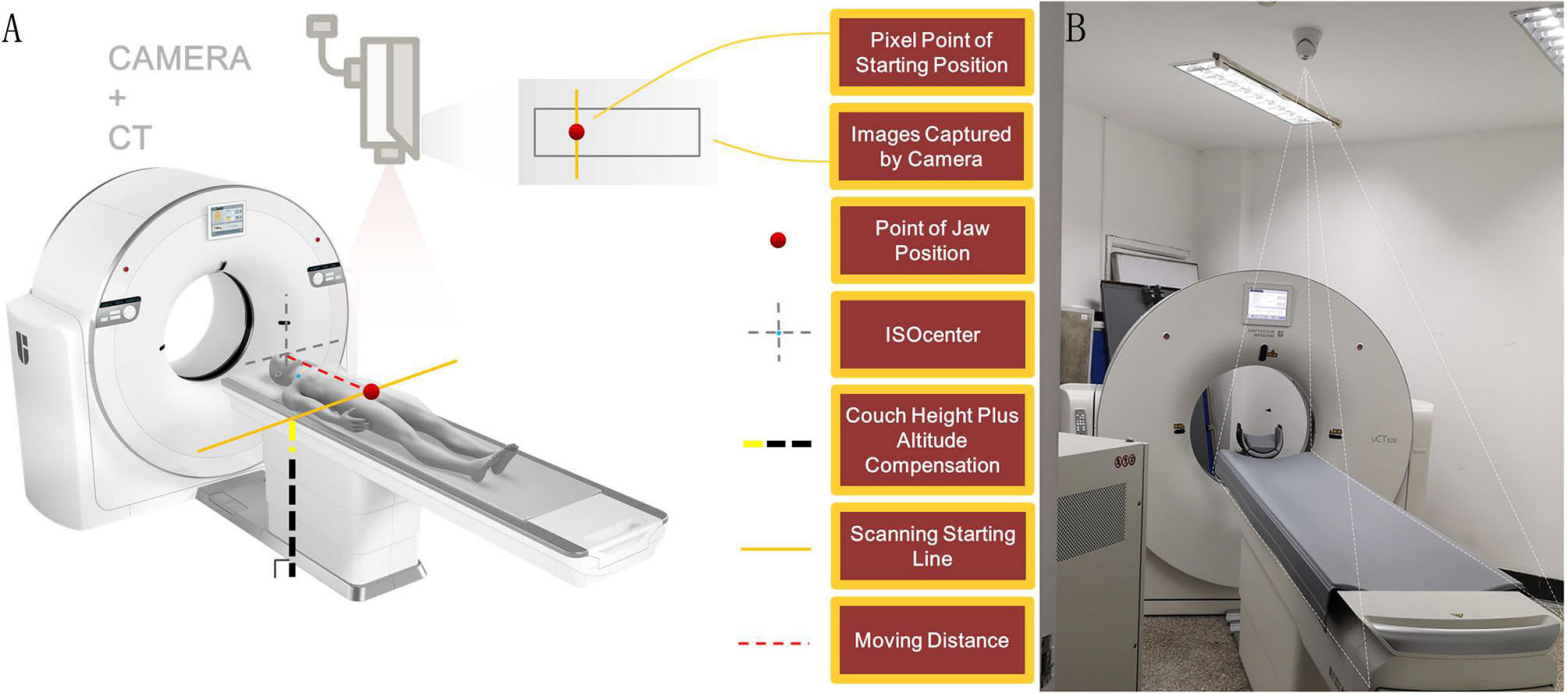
Camera-based intelligent auxiliary positioning system. (A) The working principle of the camera-based intelligent auxiliary positioning system. (B) The relocatable CT system with AI positioning technology in Wuchang Fang Cang Temporary Hospital at Hongshan Stadium in Wuchang district, Wuhan. The figures were provided through the courtesy of Shanghai United Imaging Healthcare Co., Ltd.
